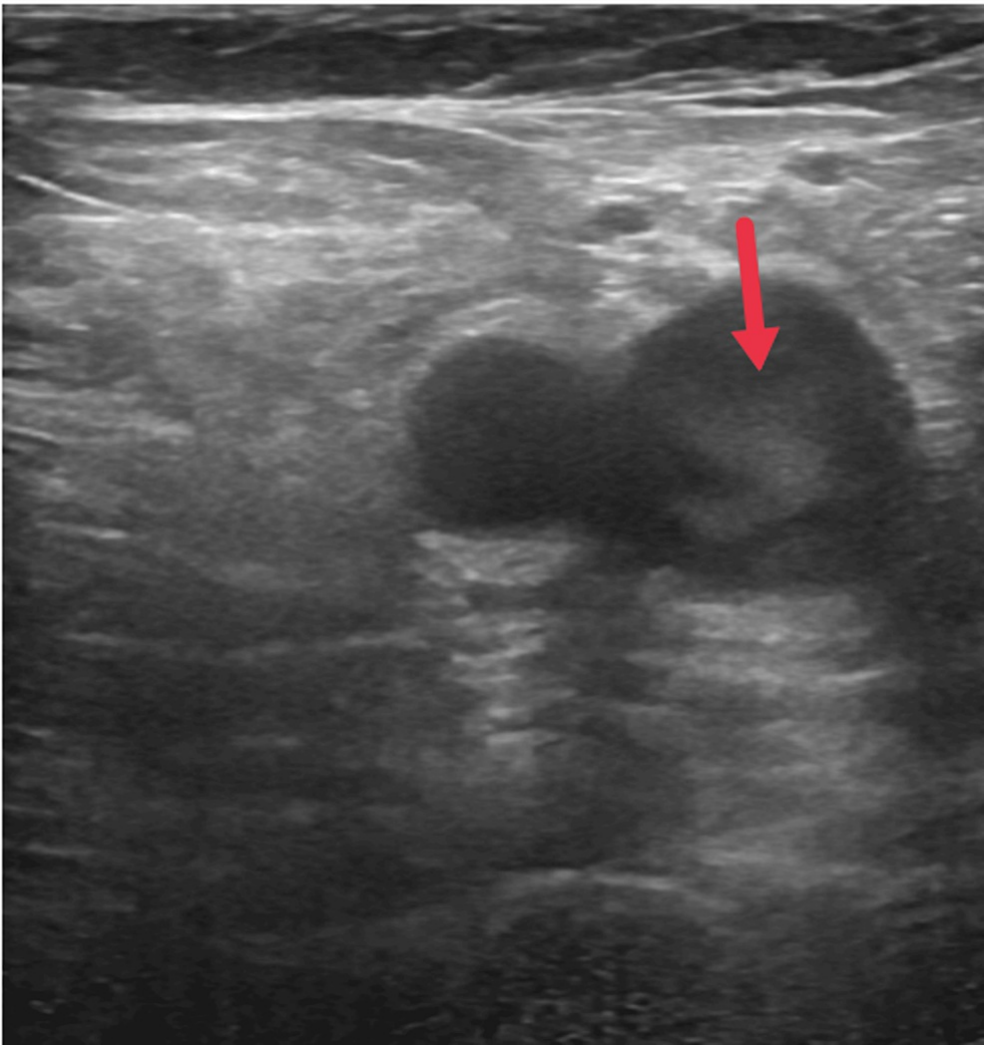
Point-of-care ultrasonography of left lower lower limb showing echogenic thrombus (red arrow) in left femoral vein.
